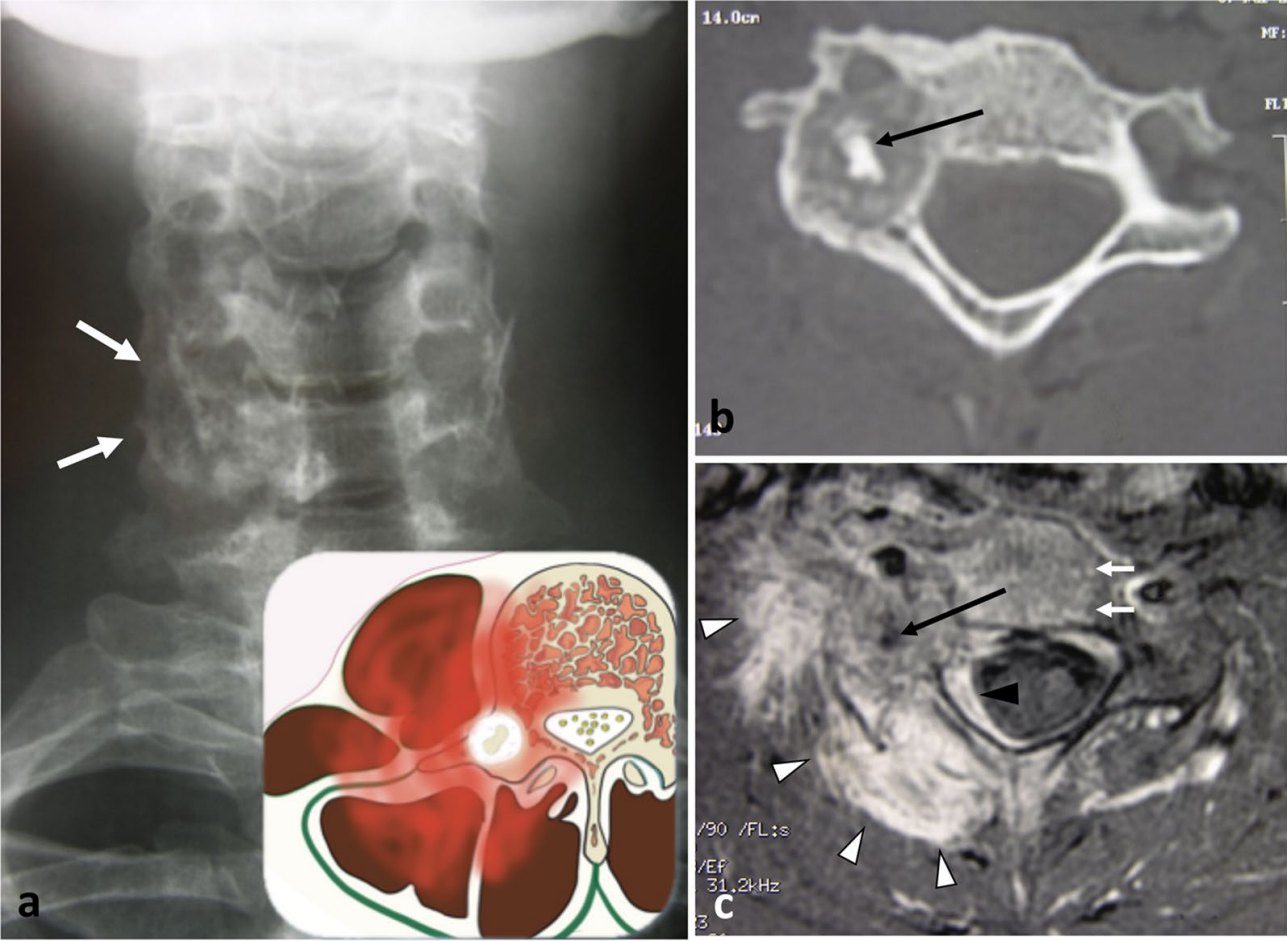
Spinal osteoblastoma* of the C6 in a 36-year-old male with intense cervical pain, worse at night, and decreased range of motion. Anteroposterior radiograph of the cervical spine (a) shows a mixed lesion of the right transverse process of C6 (white arrows). Axial CT reconstruction (b) scan shows a lytic lesion with an internal calcification (black arrow) and a rim of sclerosis (black arrowhead). Gadolinium-enhanced fat-suppressed axial T1WI (c) shows heterogeneous enhancement of the mass with associated enhancement of the surrounding paraspinal soft tissues termed ‘the flare phenomenon’ (white arrowheads). The mass invades the epidural space (black arrowhead)
